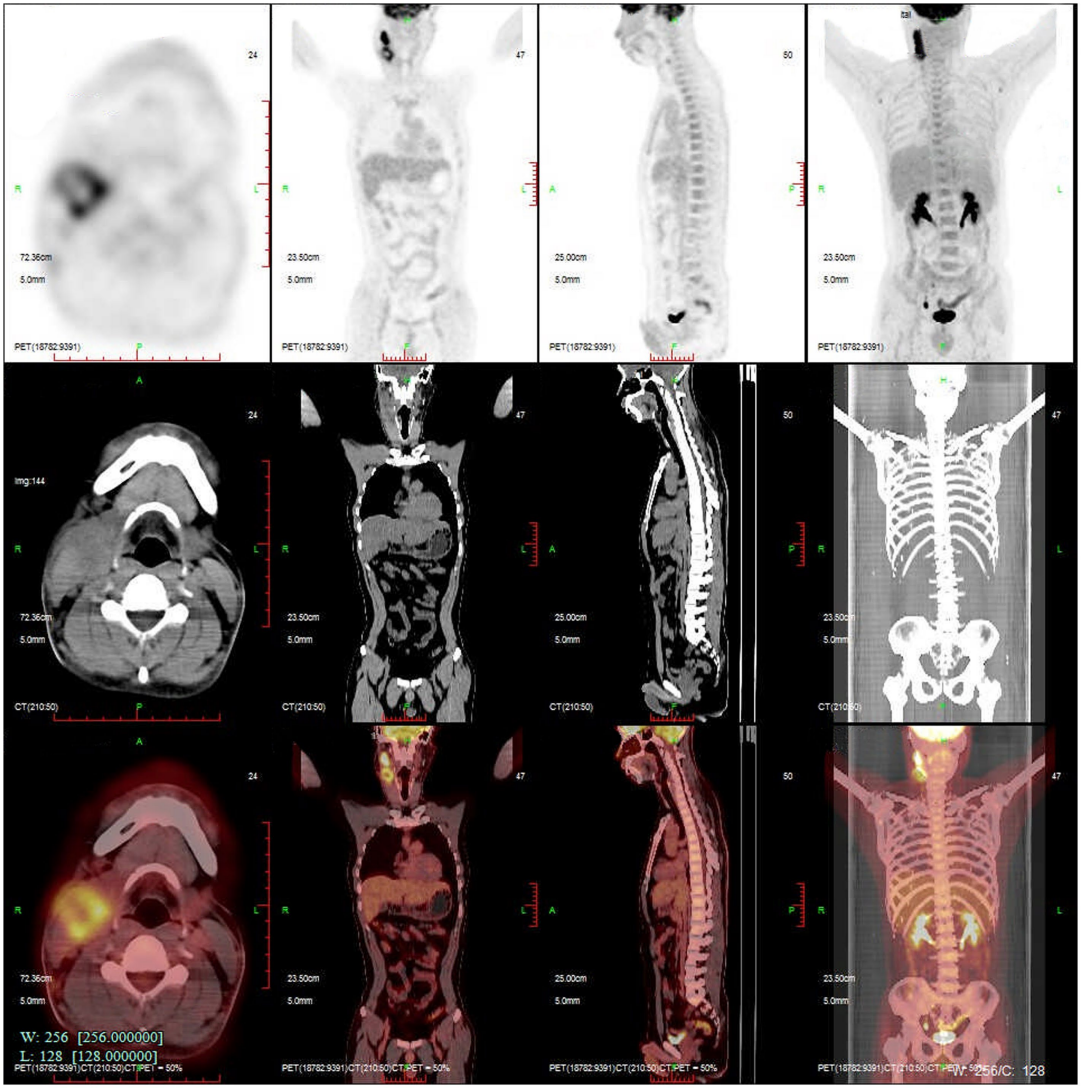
PETCT reveals several enlarged lymph nodes in the right neck with abnormally increased FDG metabolism. Malignant tumor is suspected.
